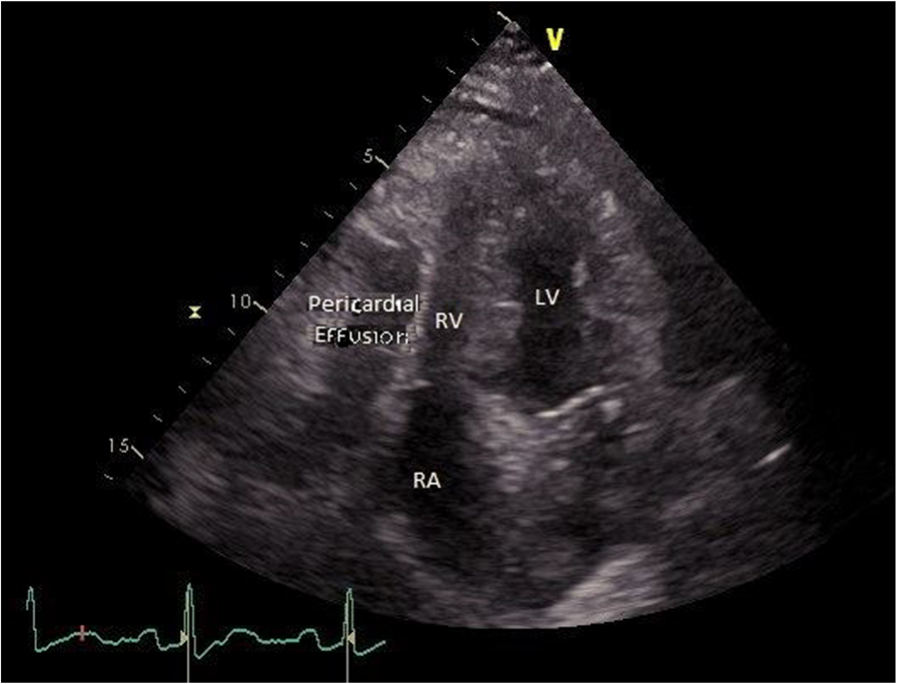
Apical four chamber transthoracic echocardiogram view. Displacement of the *RA* right atrium, *RV* right ventricle, *LV* left ventricle
